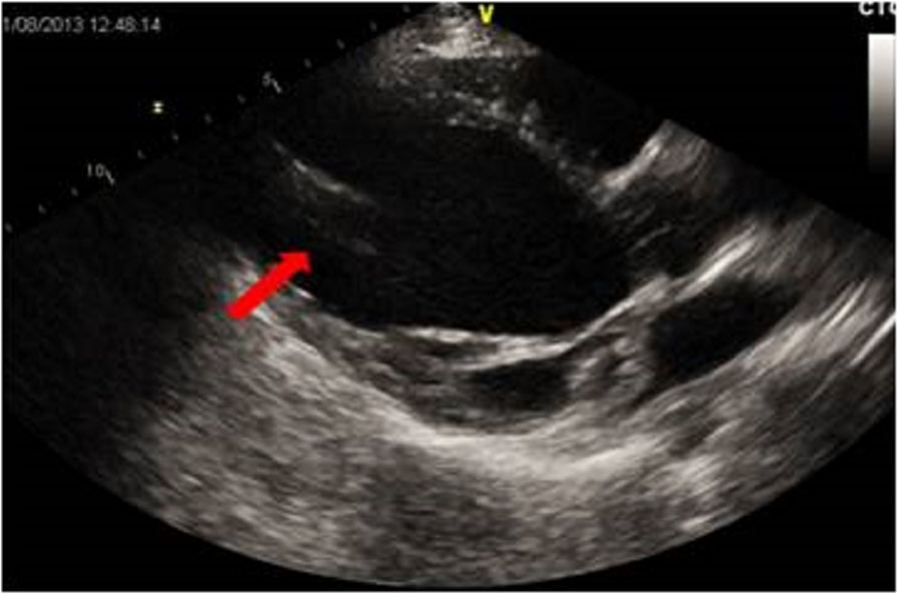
Post-implantation echocardiographic image. Cannula in the left ventricular apex (*arrow*)
